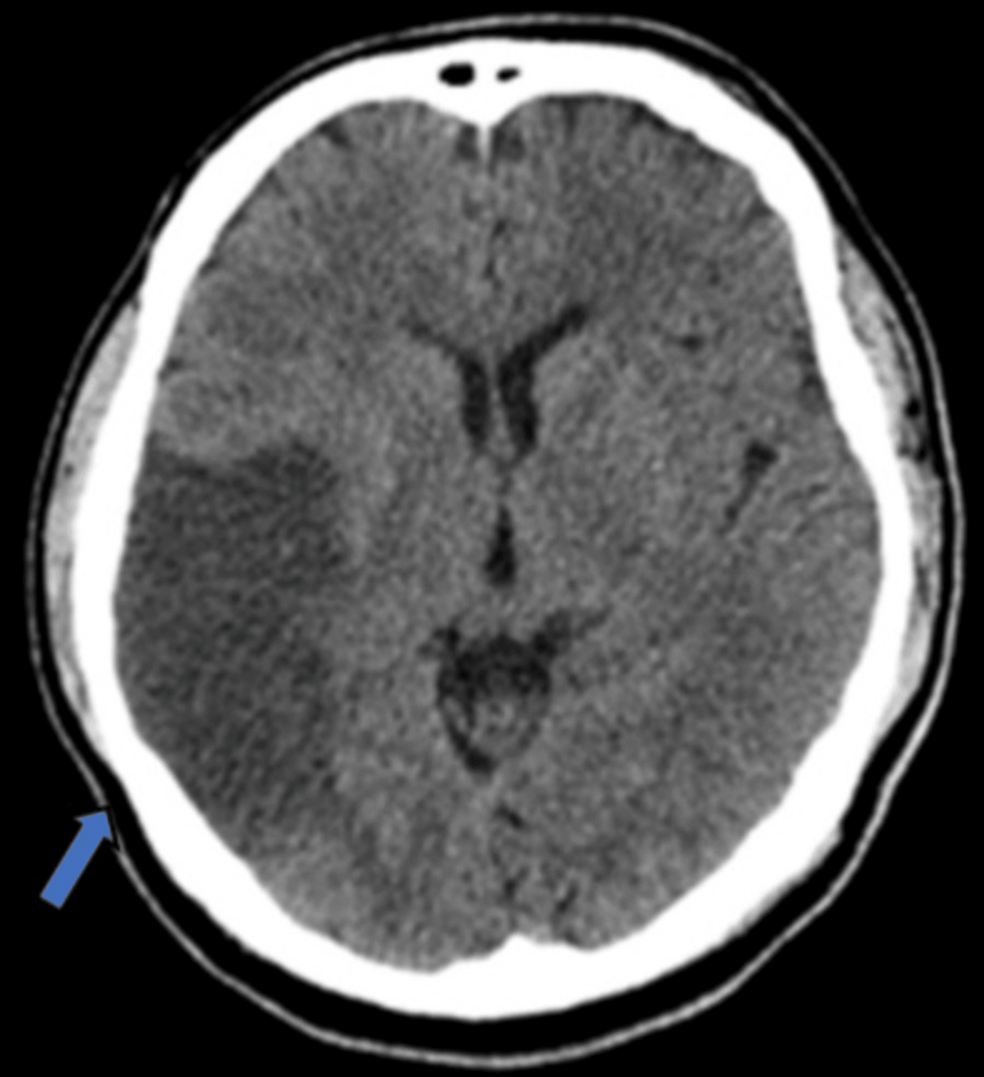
Axial non-contrast CT of the brain showing a large infarct (blue arrow) in the right middle cerebral artery territory with sulci effacement.
